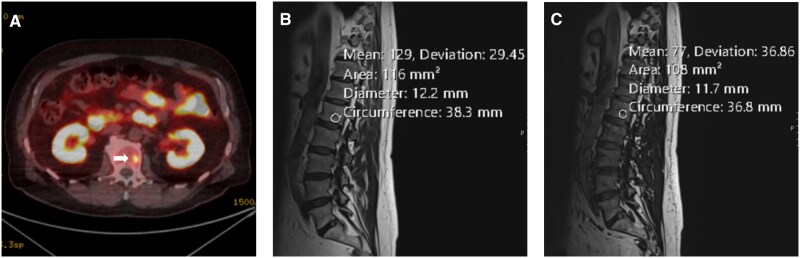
L1 vertebral body lesion (white arrow) within a 75-year-old male undergoing investigations for raised PSA. (A) PMSA PET demonstrates an avid lesion with an SUV of 9.1 (A). (B, C) Sagittal Dixon MRI sequences performed 11 months later demonstrate greater than 40% signal drop-out with mean signal intensities of 129 in-phase (B) vs 77 out-phase (C), suggestive of fat content.
